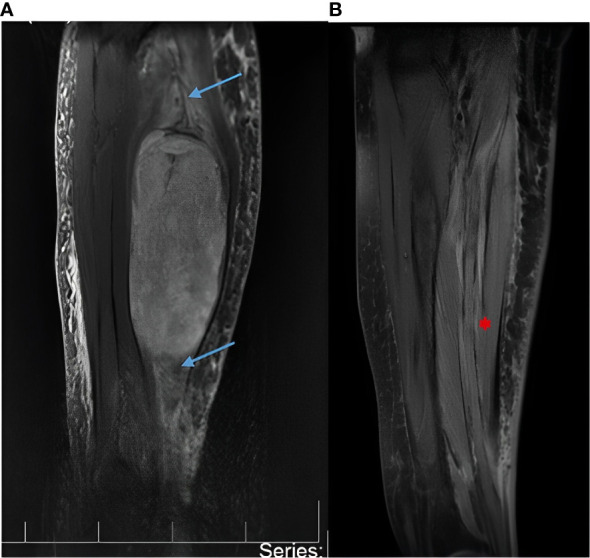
(A) Magnetic resonance imaging (MRI) with gadolinium showing a large malignant peripheral nerve sheath tumor (13x8x7cm) of the median nerve at the level of the forearm. The blue arrows show the median nerve proximal and distal to the tumor. The nerve appears enlarged due to the tumor growing in its context. The white arrow shows the tumor. (B) Postoperative MRI with gadolinium at 30 days postoperatively and every 6 months thereafter documented absence of tumor recurrence or remnants. The red arrow shows postoperative integrity of the median nerve.
